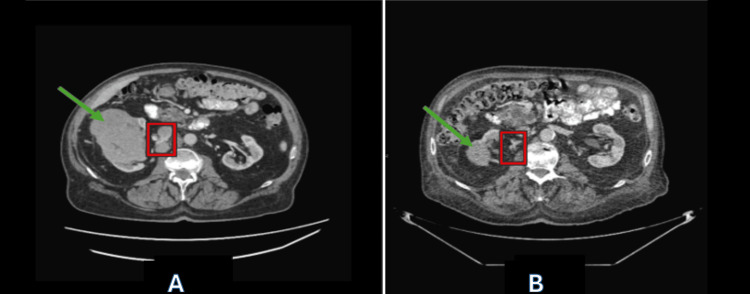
Pre- and post-treatment CT scan revealing significant regression of kidney mass and lymph node. CT scan pre-treatment (A) and post-treatment (B) showing substantial regression of the kidney mass (green arrow) and lymph node (red box).
